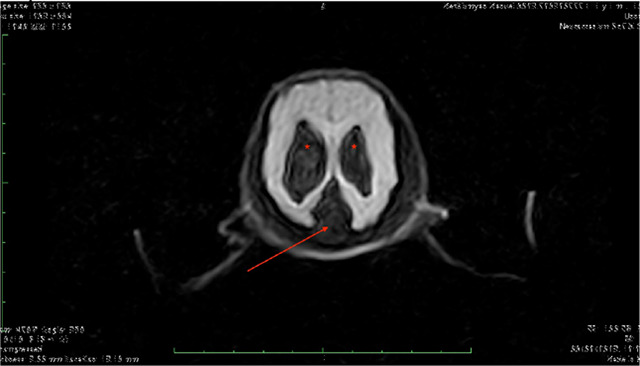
FLAIR dorsal sequence with hypointense fluid within dilatated lateral ventricles (asterisk) and a triangular quadrigeminal cyst localized between the occipital lobes (arrow)
